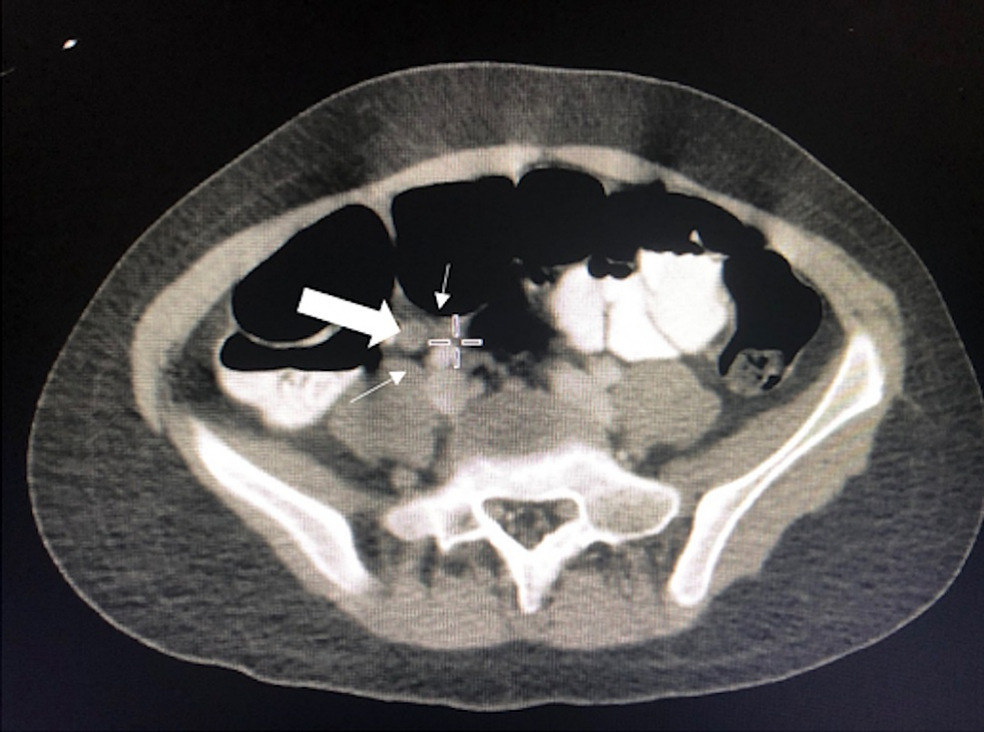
Computed tomography (CT) abdomen with contrast showing mildly inflamed appendix measuring 7-8 mm.
